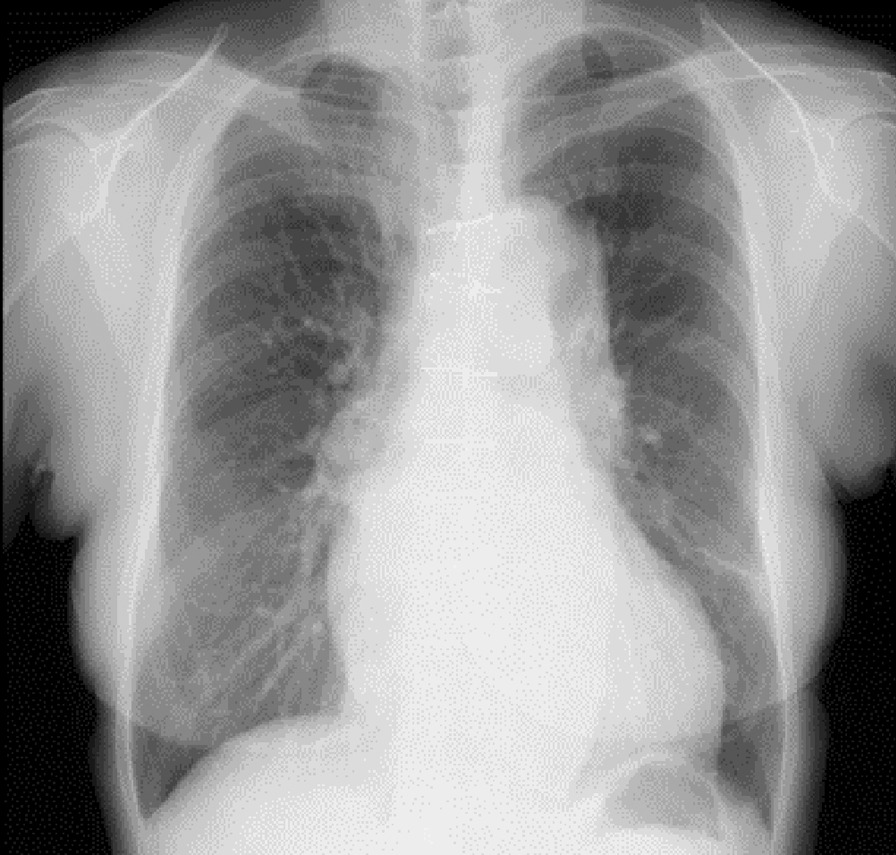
Preoperative chest X-rays. A cardiothoracic ratio of 60% is noted
